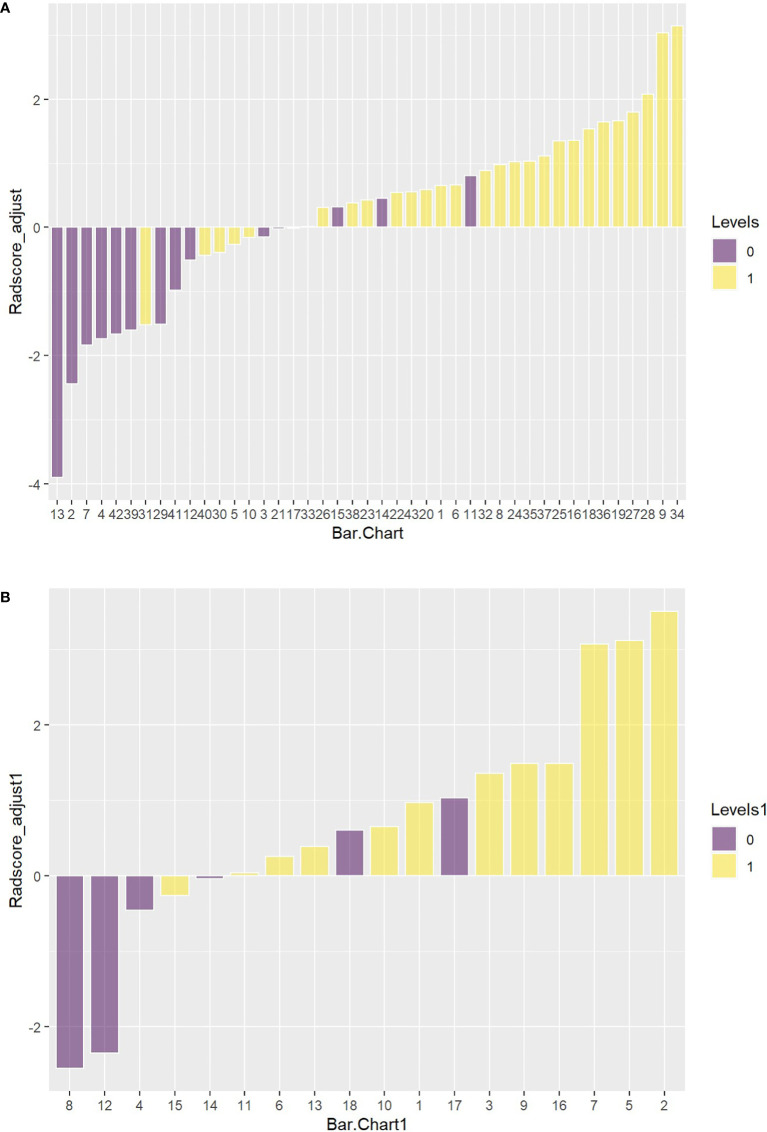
(A) The Hosmer-Lemeshow result for the PET/MRI model in the training set. (B) The Hosmer-Lemeshow results for PET/MRI model in the test set.
